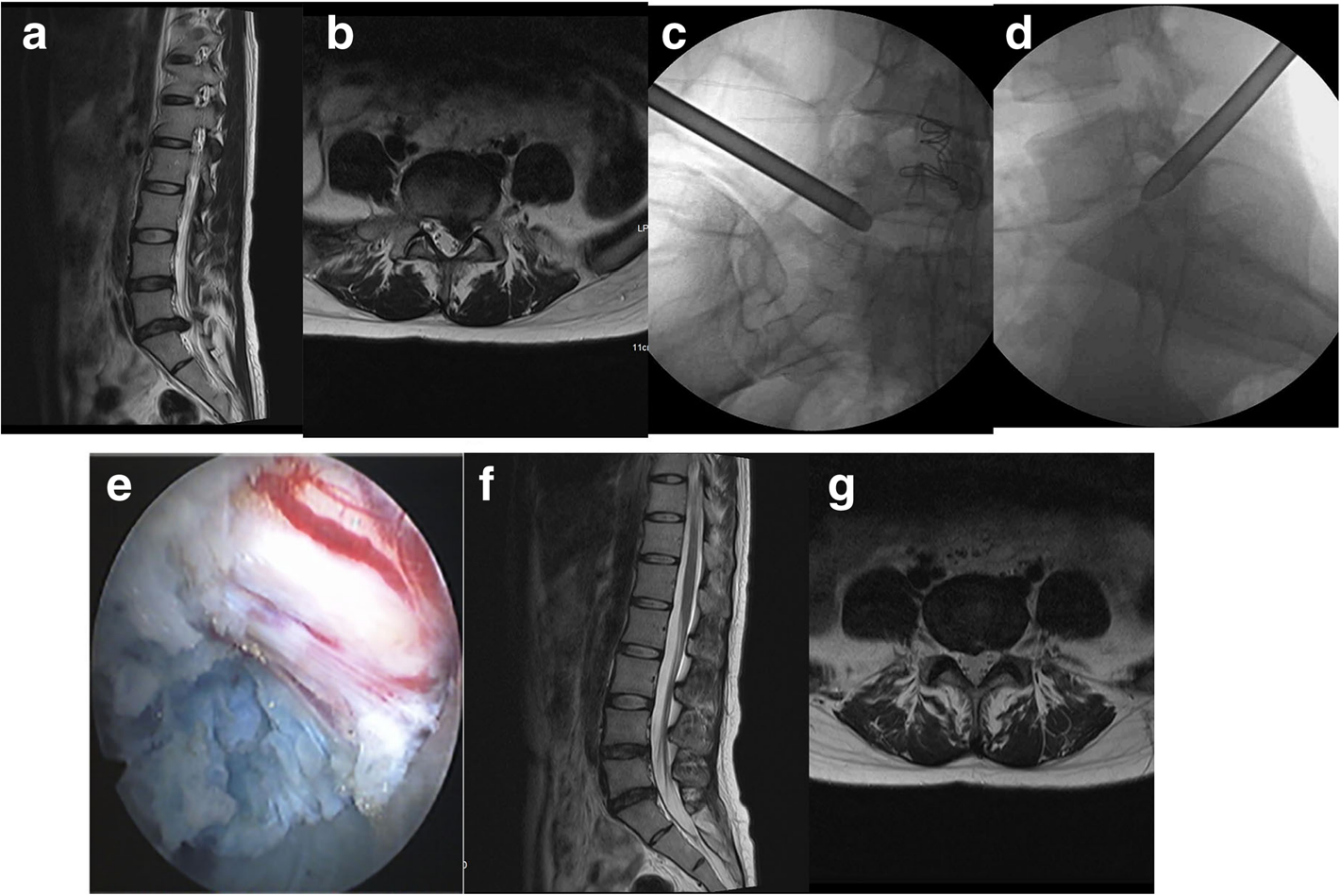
The patient was a 62-year-old male and had a protrusion in the posterior left portion of the L5/S1 interverbral discs. a MRI revealed a left L5/S1 interverbral disc protrusion in the sagittal section. b In the cross section, it revealed a protrusion in the posterior left portion of the L5/S1 interverbral discs, which compressed the left 1 sacral nerve root. c Placement of the working channel during the operation (anteroposterior film). d Placement of the working channel during the operation (lateral film). e The left five spinal nerve root was exposed during the operation. f After the operation, MRI revealed no significant protrusion in the L5/S1 interverbral discs. g After the operation, in the transverse section, it revealed no significant protrusion in the L5/S1 interverbral discs, and the nerve roots were not compressed
